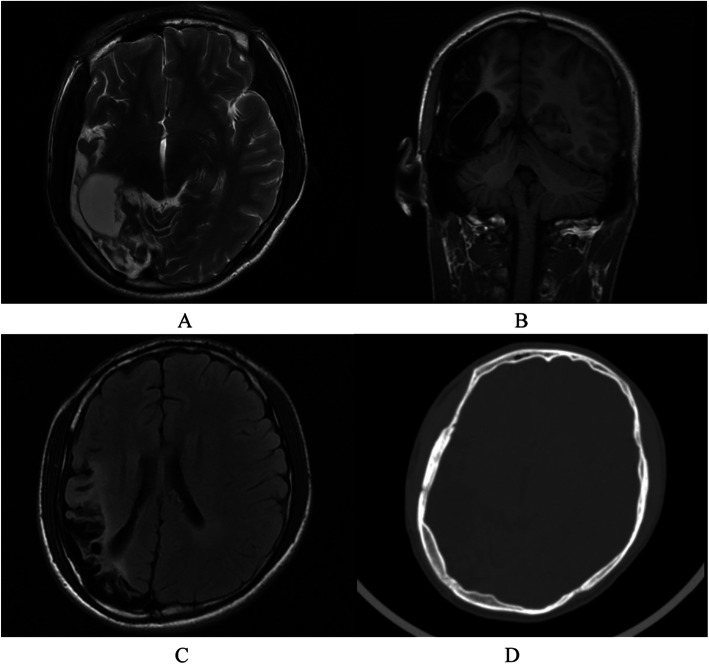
A 15-year-old teenager with DDMs (Case6). Axial T2-weighted imaging showed (a), coronal T1-weighted (b) and axial FLAIR MR images, demonstrating volume loss of the right-sided temporal, parietal and occipital lobe and sulcal enlargement, midline structure shifted to the affected side. Dilated temporal horn of the lateral ventricle was shown to be significant. Axial CT image (d) demonstrated thickening of ipsilateral cranial bones diploe
